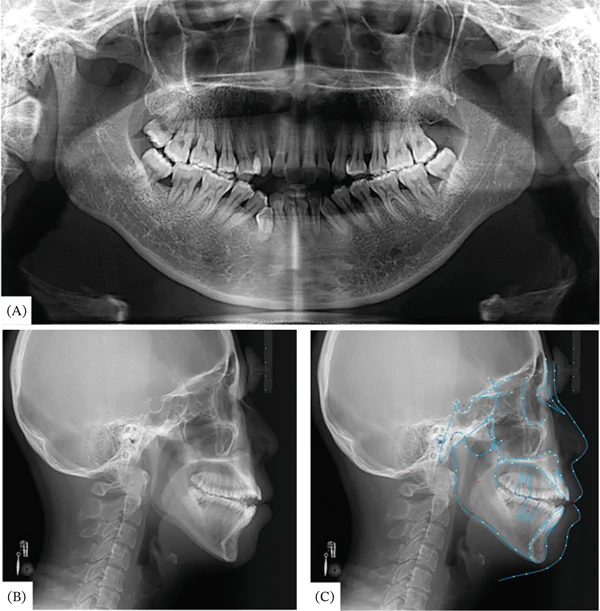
Pretreatment radiographs. (A) Panoramic radiograph, (B) lateral cephalogram, and (C) lateral cephalogram tracing.
